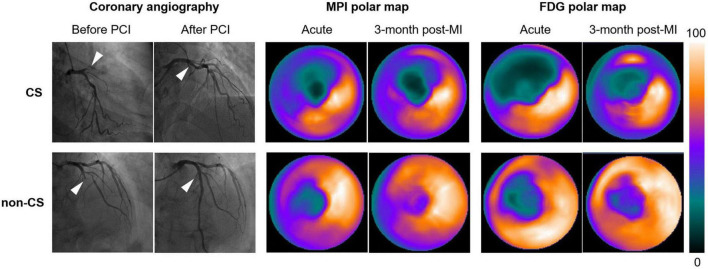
Representative ^99m^Tc-sestamibi/FDG images of STEMI patients with and without CS following successful PPCI in the LAD (white arrows). Polar maps identified larger extent of impaired myocardial perfusion and metabolism in patients with CS both in the acute phase and in the convalescent phase. CS, cardiogenic shock; PPCI, primary percutaneous coronary intervention; LAD, left anterior descending artery; TFD, total FDG uptake deficit.
